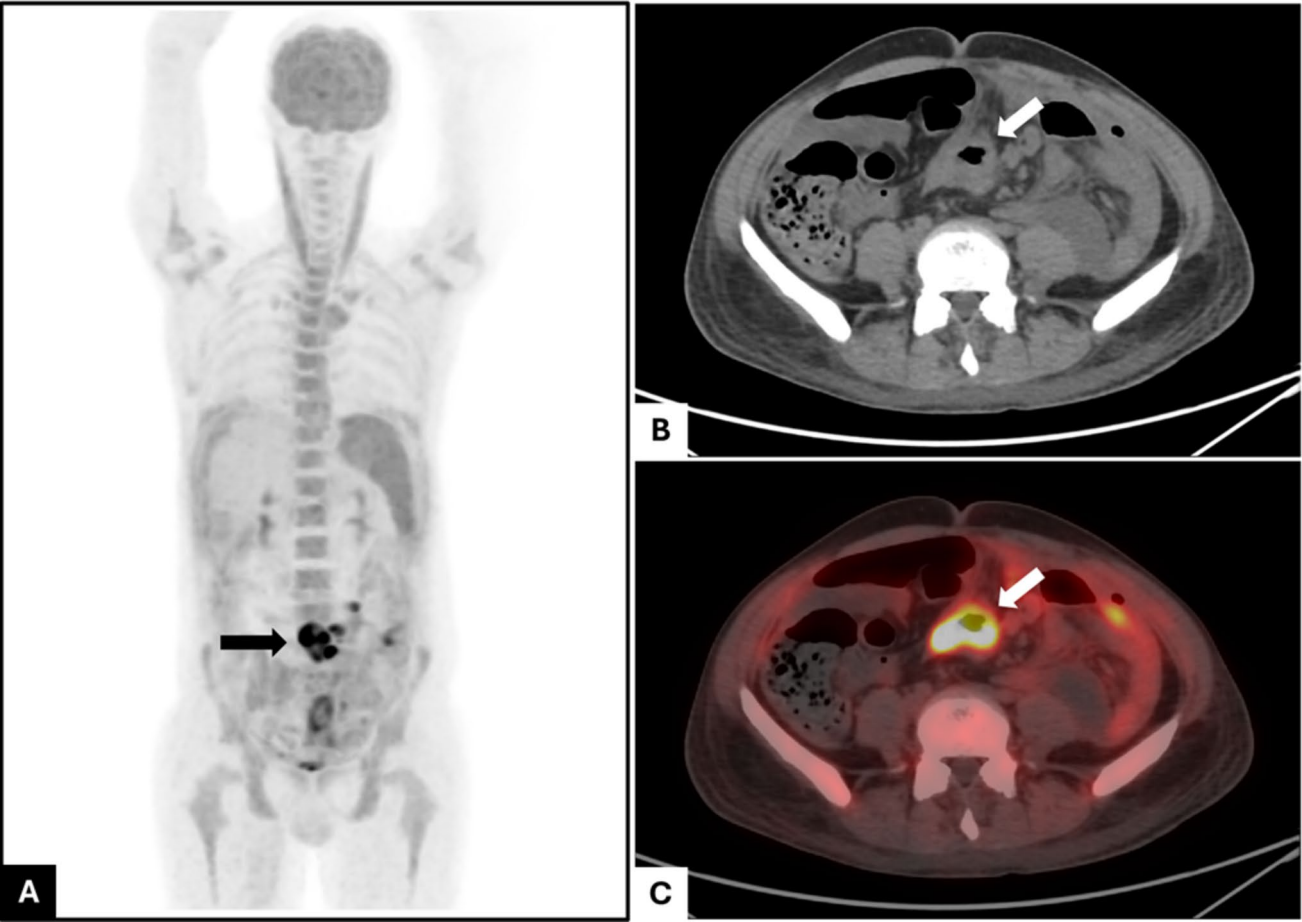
PET-CT whole body A Maximum intensity projection (MIP) image showing a focus of increased tracer uptake in small bowel (back arrow; coronal plane). B, C CT and PET-CT fusion images showing circumferential thickening in small bowel with increased 18F-FDG uptake (white arrow, transverse plane)
